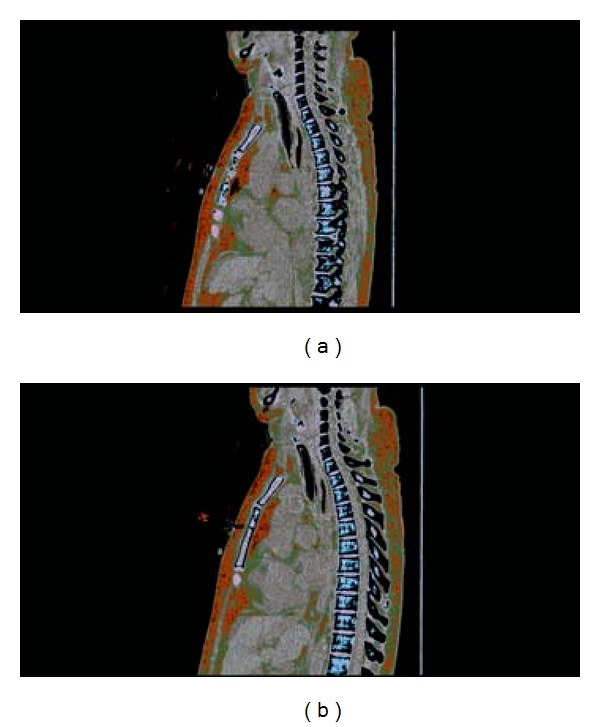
CT thorax, inspiration (a) and expiration (b), the figure tracheomalacia protocol; demonstrates near complete collapse of the distal trachea during expiration.
